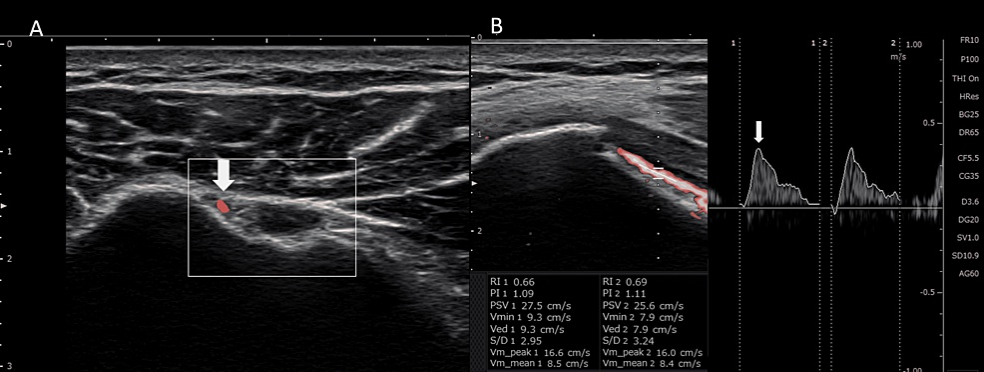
Ultrasonography A) Transverse color Doppler image of the bicipital groove with the anterior humeral circumflex artery (arrow). B) Longitudinal-pulse Doppler image of the anterior humeral circumflex artery with peak systolic velocity (arrow).
